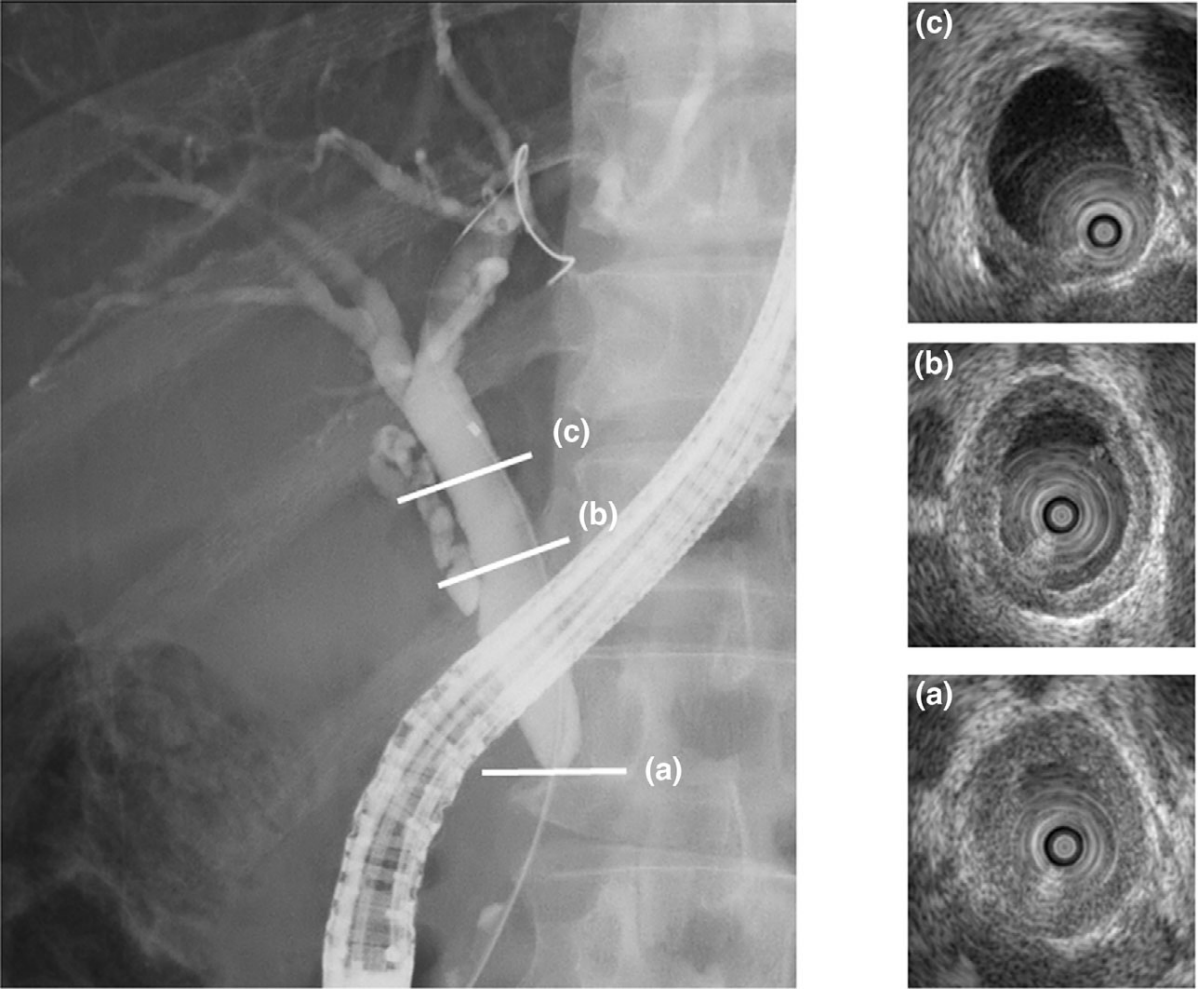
Intraductal ultrasonography (IDUS) findings of immunoglobulin G4 (IgG4)‐related sclerosing cholangitis (IgG4‐SC). Endoscopic retrograde cholangiography showing intrapancreatic strictures. (a) IDUS reveals wall thickening in the intrapancreatic stricture. IDUS findings include circular‐symmetrical wall thickening with smooth inner and outer margins. (b) IDUS demonstrates wall thickening in the middle bile duct at a nonstricture site. (c) IDUS shows wall thickening in the hilar bile duct at a nonstricture site.
